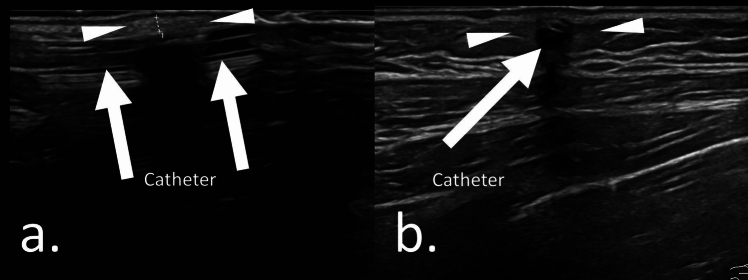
Case 19: Ultrasonographic findings in a 5.7-year-old boy with symptoms of erythema without unplanned CVC extraction. The patient was not diagnosed with complications associated with the tunneled catheter. The onset of symptoms was 112 days after catheter placement. Catheter removal was performed 154 days after symptom onset. a. Ultrasonography showing a hyperechoic area around the catheter (solid arrowheads) b. Ultrasonography does not show fluid collection around the catheter (solid arrowheads). Fluid collection and hyperechoic foci are absent.
